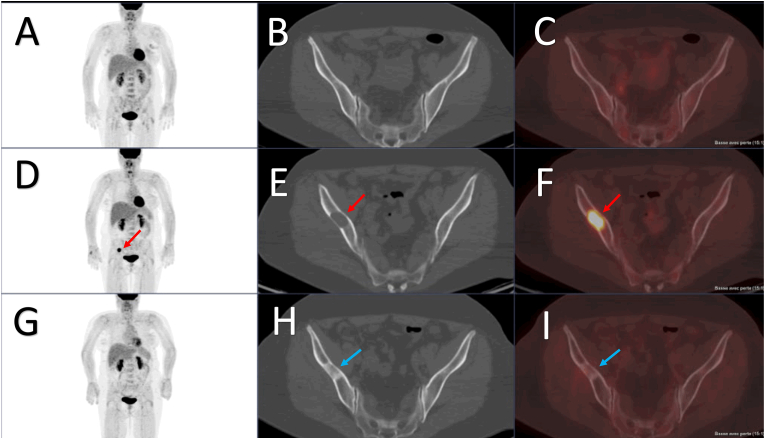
A 56-year-old woman with NST BC, grade 2, ER+, PR−, HER2- (luminal B) with confirmed axillary lymph nodes involvement was submitted to primary surgery. Baseline PET/CT staging performed after surgery showed no pathological [18F]FDG uptake (A, whole body PET MIP image, and B, C, axial CT and PET/CT fusion images of the iliac bones). Two years later, the patient was referred for a new [^18^F]FDG PET/CT examination because of isolated CEA elevation. A single lytic metastasis of the right iliac bone (red arrows) was detected (D, whole body PET MIP image; and E, F, axial CT and PET/CT fusion images of the iliac bone). The patient was treated with stereotaxic radiotherapy and targeted therapy. Six months later, PET/CT showed a complete metabolic response (G, H, I). The focus of the right iliac bone was replaced by a non-[^18^F]FDG-avid osteosclerosis (blue arrows) suggestive of a healed lesion (H and I, axial views of CT and PET/CT fusion image).
